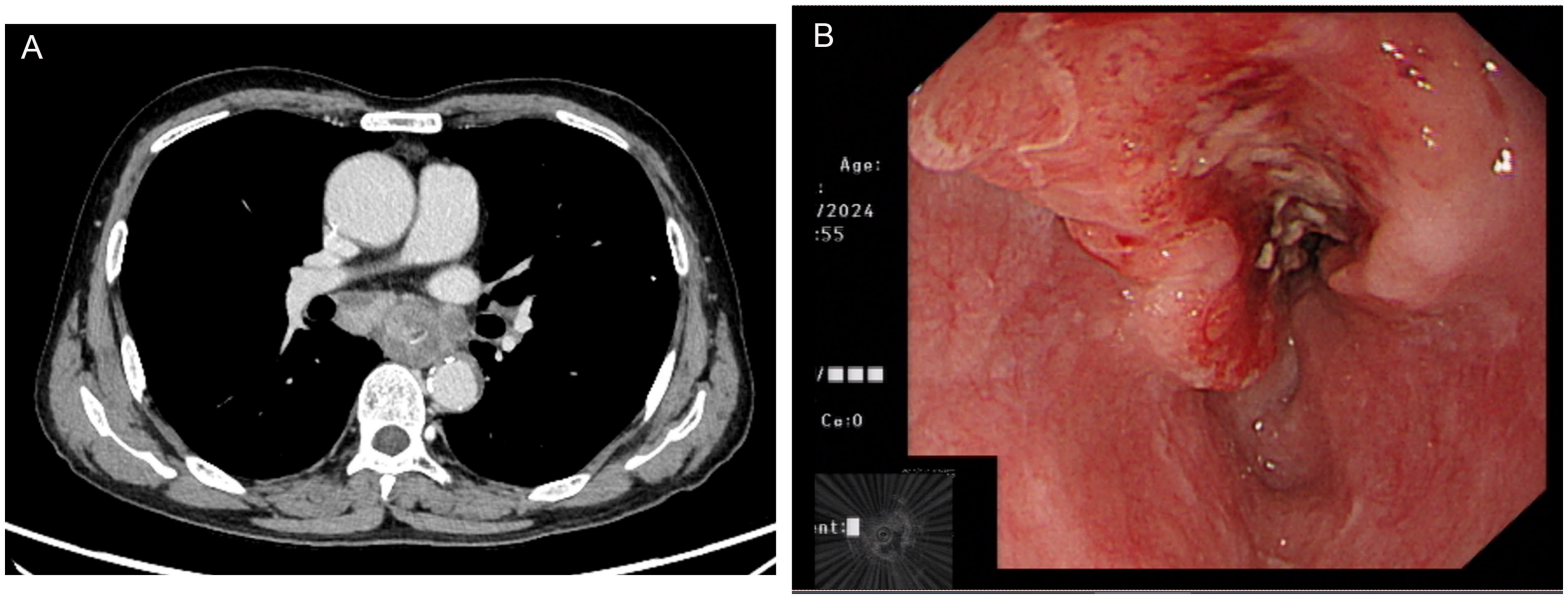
Chest computed tomography (A) and endoscopic ultrasonography (B) showed esophageal squamous cell carcinoma in the mid-chest area.
